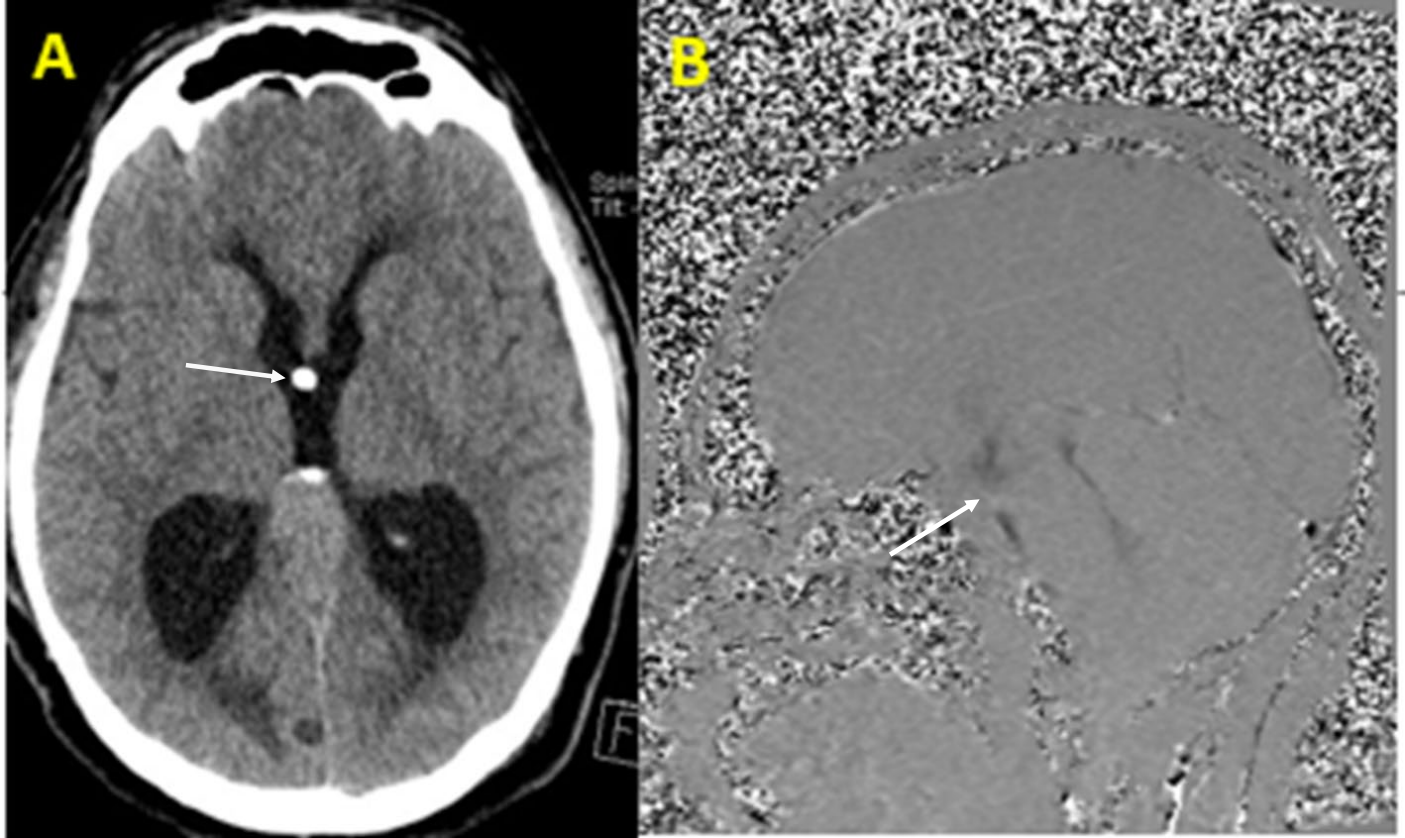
A Postoperative CT brain showing the EVD tip (white arrow) passing through foramen of monro. B A flow-sensitive MRI showing patent ventriculostomy ostium (white arrow) black signal in MRI with CSF flowmetry due to turbulance of CSF across the ventriculostomy site
